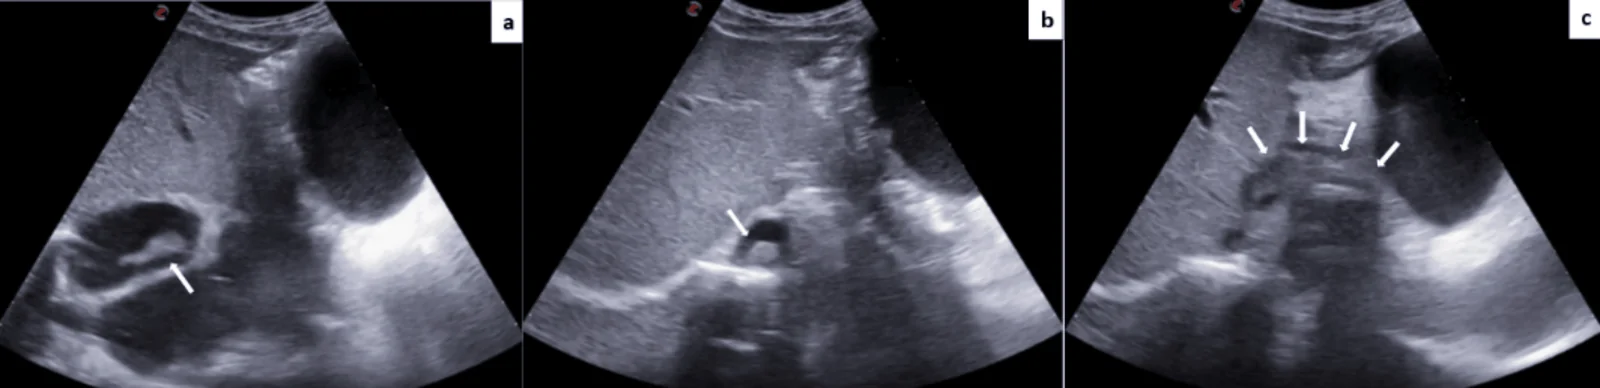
Focused ultrasound following pigtail placement and drainage (a) Linear echogenic structure (white arrow) within the right atrial cavity, representing the apposed walls of the decompressed collection, subxiphoid four-chamber view; (b) rounded echogenic structure (white arrow) within the inferior vena caval lumen, transverse subcostal view; (c) the same structure in longitudinal section (white arrows), demonstrating continuity with the abdominal component and absence of fragmentation
